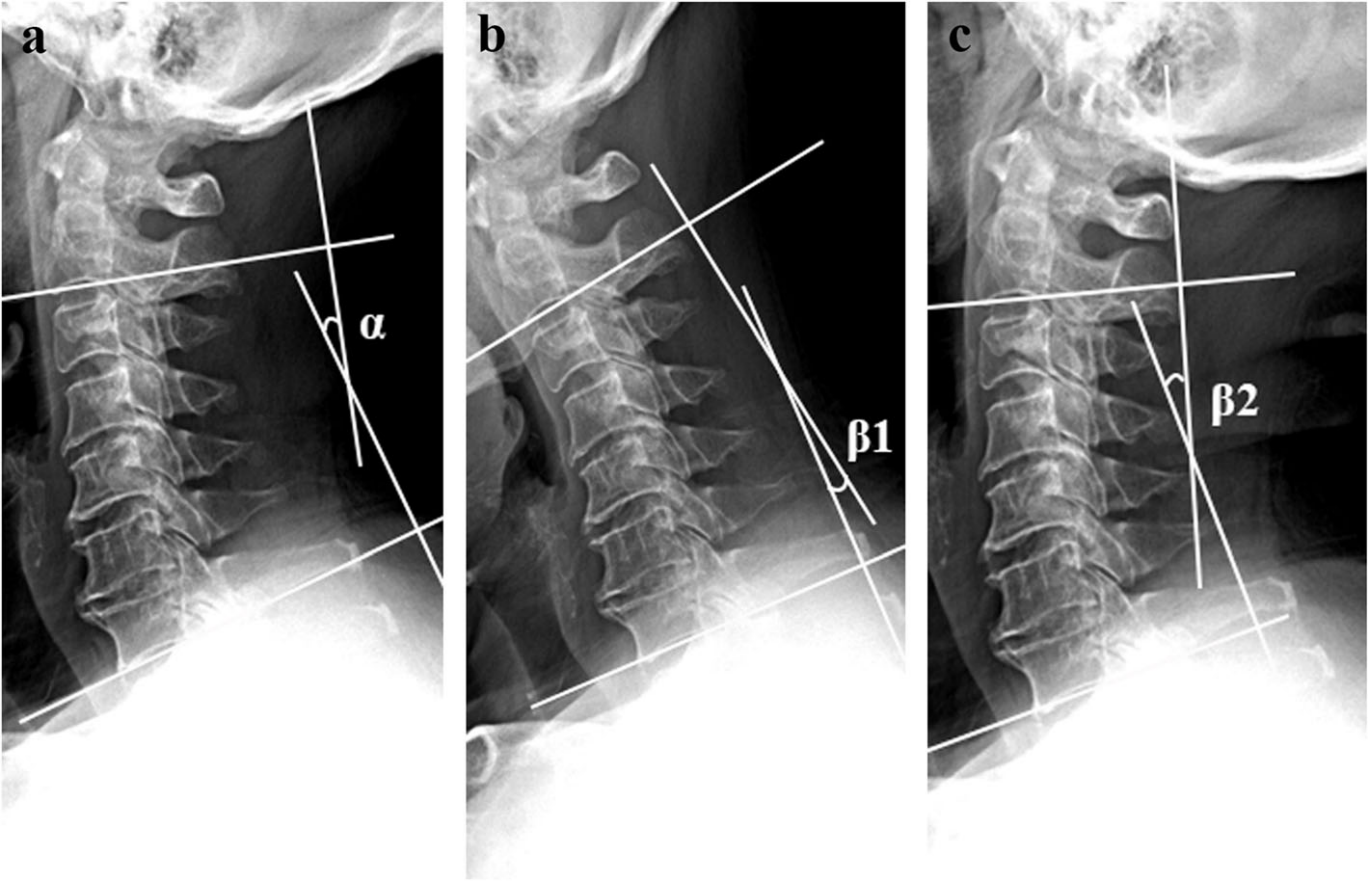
The C2-C7 Cobb (α) angle was defined as the angle between the line parallel to the inferior endplate of C2 and C7 (a). The cervical range of motion (ROM) was defined as the sum of C2-7 Cobb angles measured on flexion-extension lateral radiographs (b and c). ROM = β1 + β2
